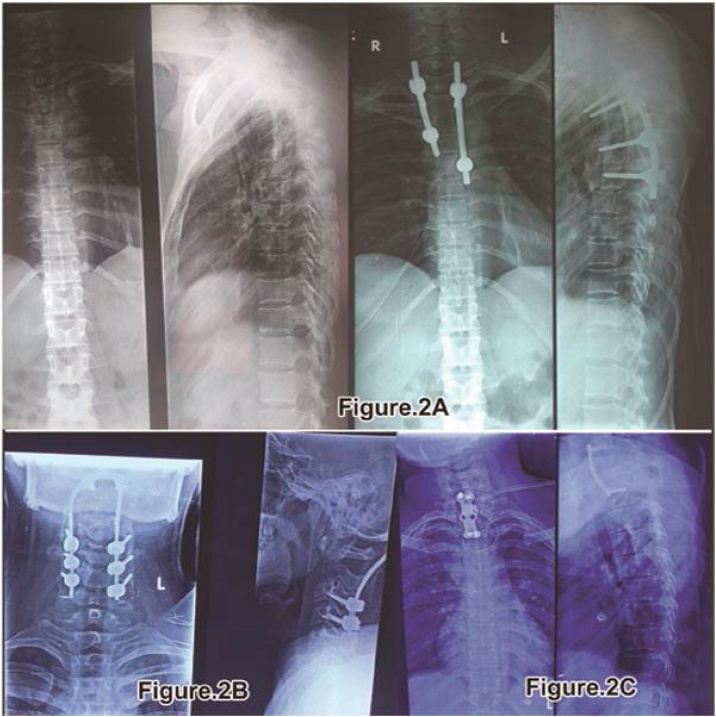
Post-operative xray images showing the surgical intervention the patient underwent. A:Post-operative xray showing rib graft in situ and posterior fixation done after recurrence. B:Post-operative xray showing Lateral mass screw fixation at C2 level. C:Post-operative xray showing long cervical plate in situ after corpectomy C7-D1.
